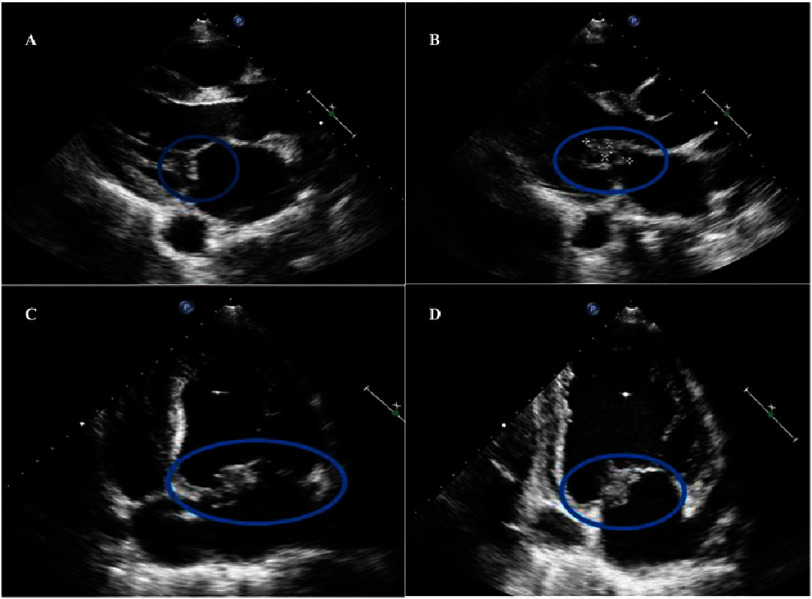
(A and B) Transthoracic echocardiogram, parasternal long axis view showing thickening of the leaflets and a mass attached to the anterior leaflet of the mitral valve measuring 2.94 × 0.88 cm. (C and D) Apical 4 and 2 chambers; mass on the anterior leaflet.
